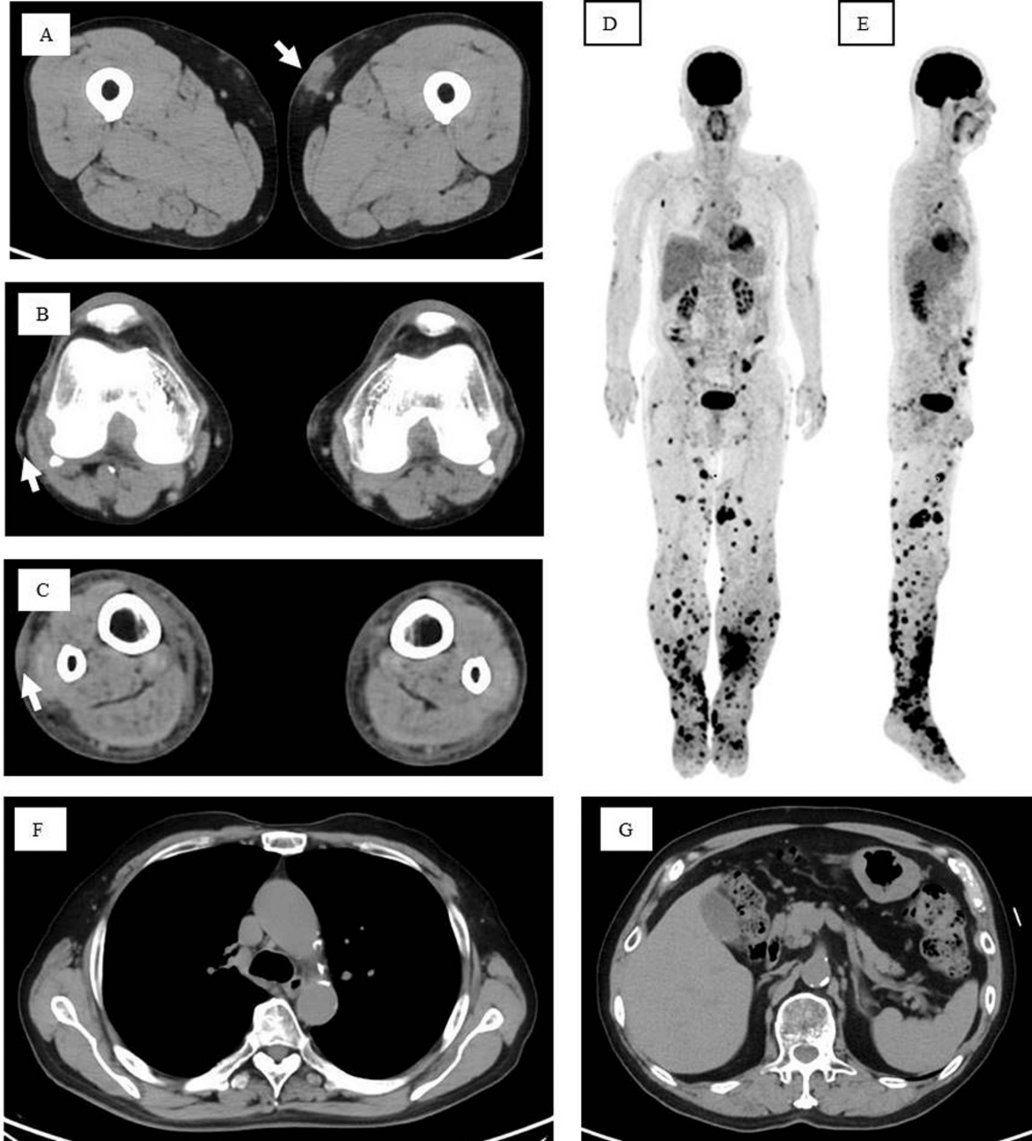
Imaging analyses A-C: Computed tomography (CT) images of the legs in March in the second year of onset. Tumor in the left upper leg (A), right knee joint (B), and distal aspect of the right lower leg (C). The tumors are indicated by the white arrows. D, E: Positron emission tomography-CT (PET-CT) images in May in the second year of onset. Anterior (D) and side (E) aspects of PET-CT images. The substantial uptake of ^18^F-fluorodeoxyglucose (FDG) is distributed on the surface of both legs (compare anterior and side aspect images); conversely, FDG accumulation in the brain and other internal organs appears physiological. F, G: Chest and abdominal CT images showing no pathological lymph node enlargement or internal organ lesions. Slices at the pulmonary hilus (F) and beginning of the celiac trunk (G) were chosen as representatives.
